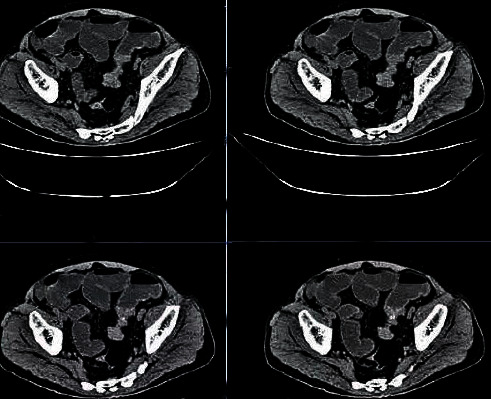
CT image of a 52-year-old male patient complaining mainly about loose stools, changes in excrement, and occasional stomachache for more than one year.
